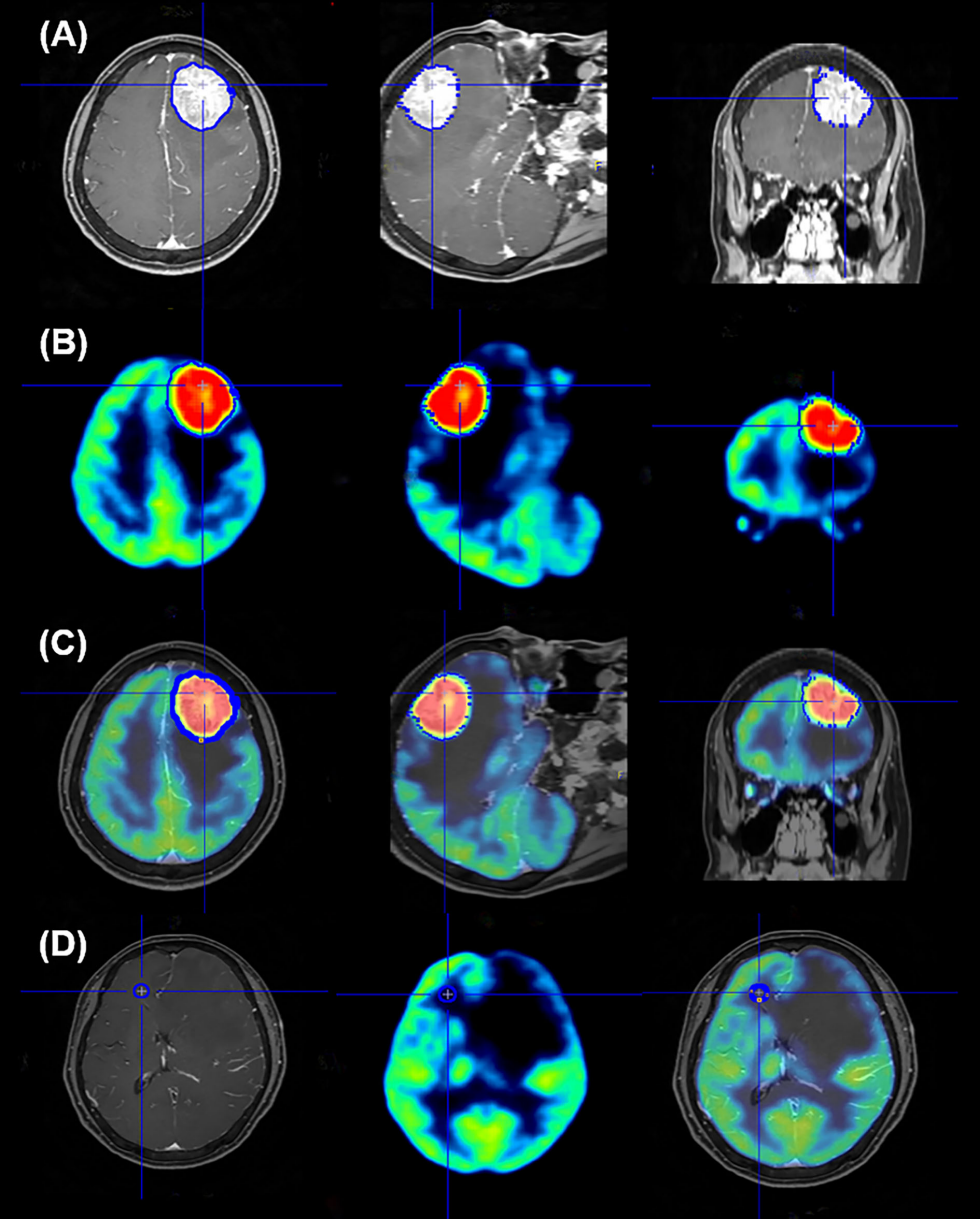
Representative image of region of interest setting for quantification analysis of ^18^F-FDG PET data. The volume of interest (VOI) was automatically delineated to brain lesions on MRI (A); this edge of VOI was projected onto the PET image (B) and the VOI is seen in the image of the PET and MR fusion (C). To set the reference area, the ROI is confirmed by setting the circular shape on the frontal white matter on the opposite side of the metastatic brain lesion and projecting it on the PET and PET/MR fusion images (D).
